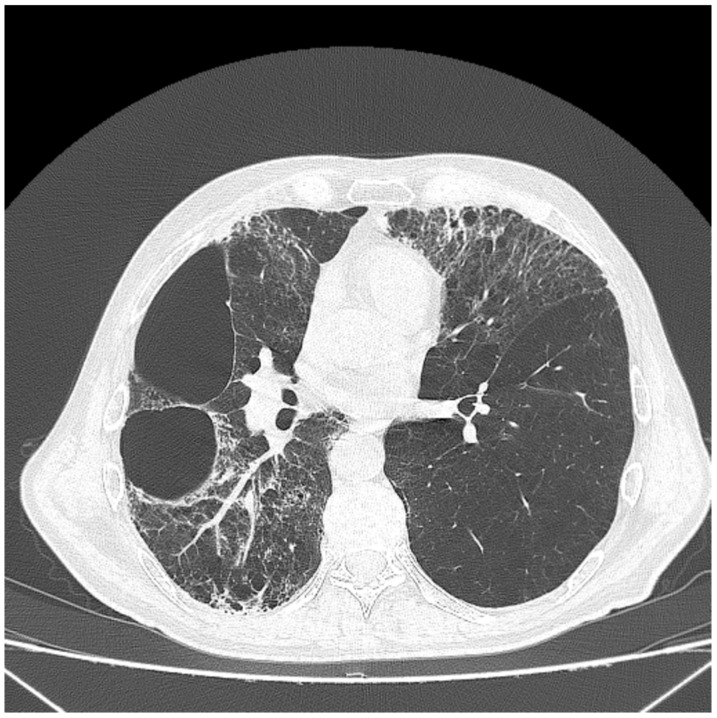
CT evaluation—2020—during acute viral infection indicated bilateral pneumonia, with ‘honeycomb’ lesions, which were confluent in the two big bullae on the right.
